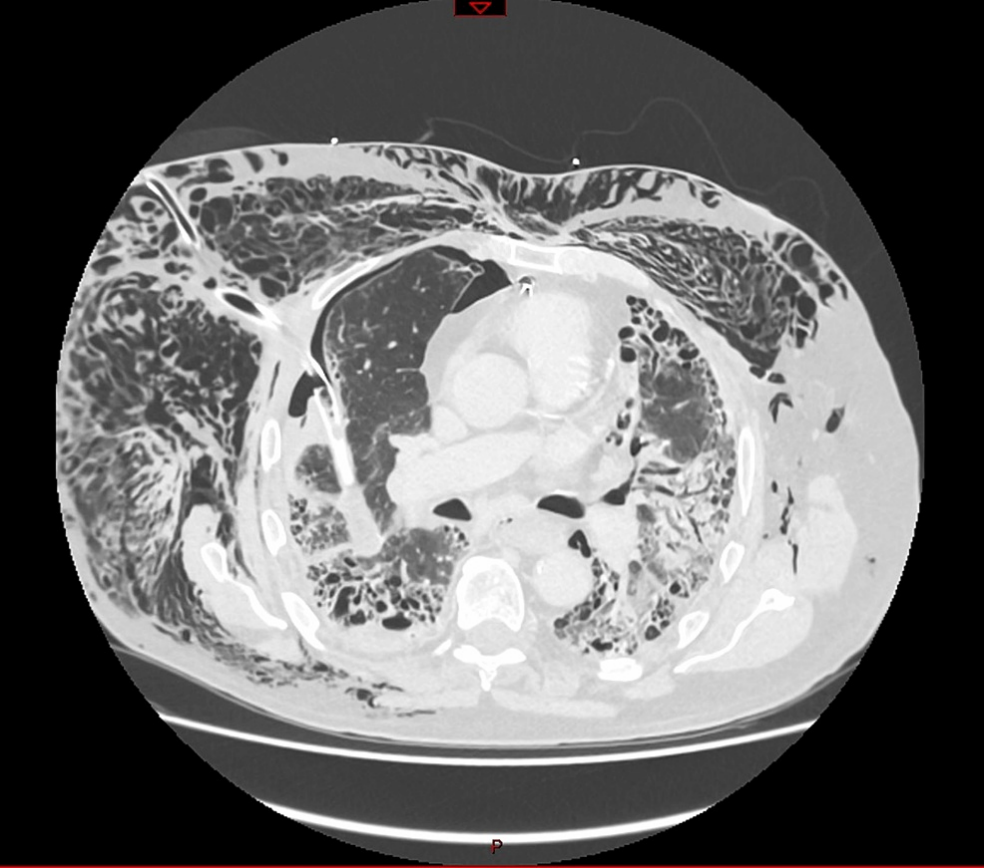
Chest drain intraparenchymal of the right lung. Chest CT scan with contrast. Chest drain penetrating the right lung.
